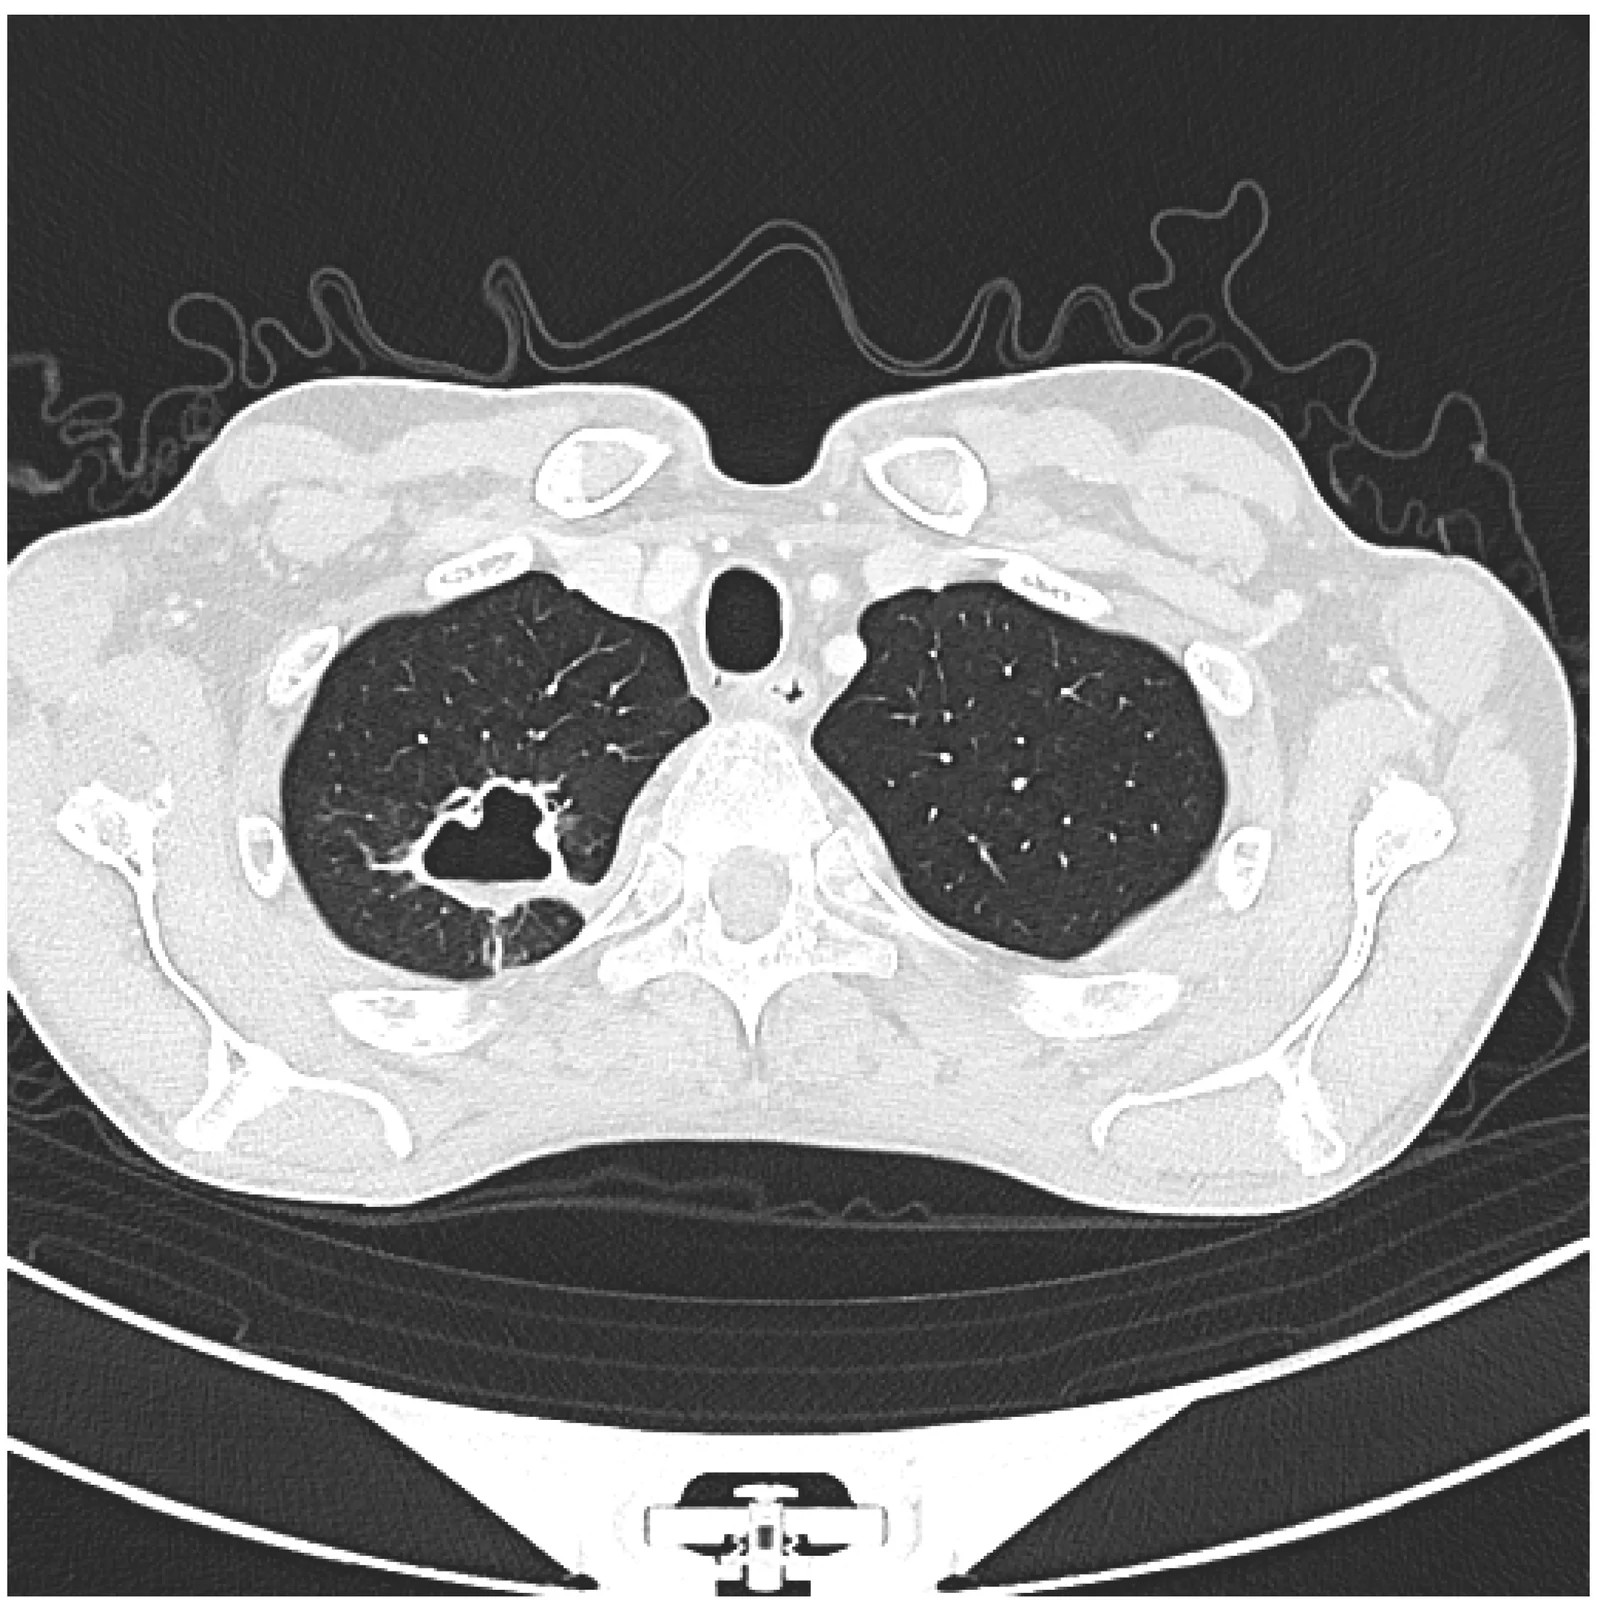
HRCT, axial plane. CT scan shows the cavitary radiologic pattern of MAC infection in a male patient. A single cavitated lesion can be noted in the right upper lobe. This lesion could be very difficult to differentiate from other type of cavitary lesions and notably from a TB lesion.
